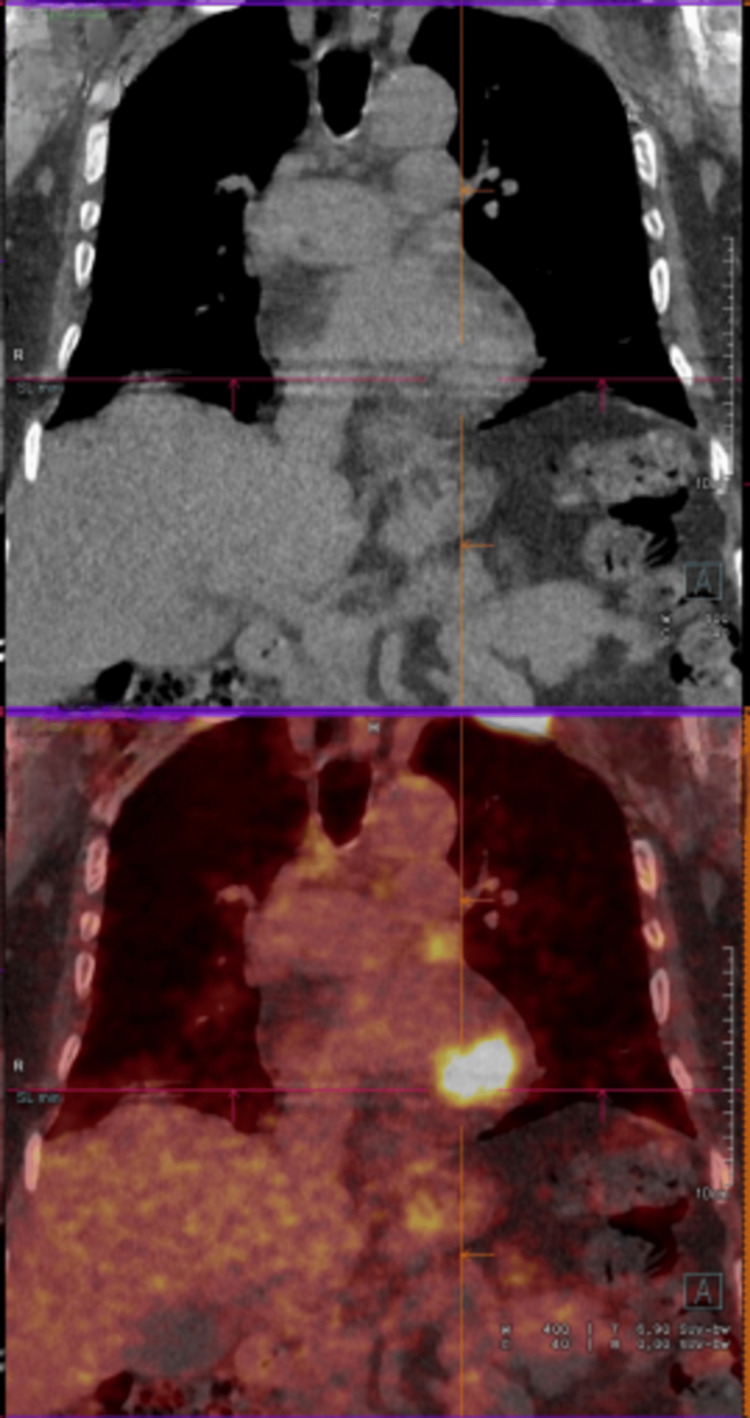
PET-FDG with an area of hypermetabolism in the left ventricle in the coronal section.
